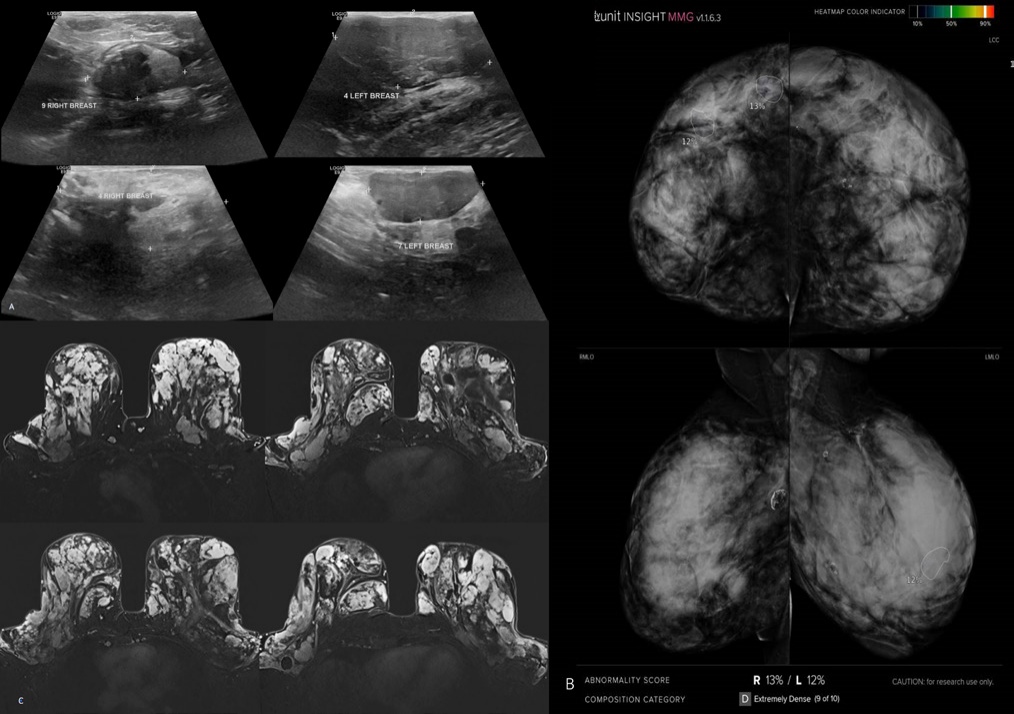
Idiopathic gigantomastia of a 34-year-old female with extremely dense breasts (ACR d). A, Ultrasound images of both breasts showed multiple solid masses, some of them showed indeterminate features of heterogeneous texture and lobulated outline (BI-RADS 3). B: four view mammograms scanned by the AI algorithm showed low scoring of malignancy at both breasts (right, 13% and left, 12%). C, MR examination, axial subtraction post contrast images; showed both breasts studded by multiple variable-sized solid masses characteristic of adenomas. Biopsy from the ones that showed high vascularity revealed simple fibroadenoma. The case was a true negative for AI
